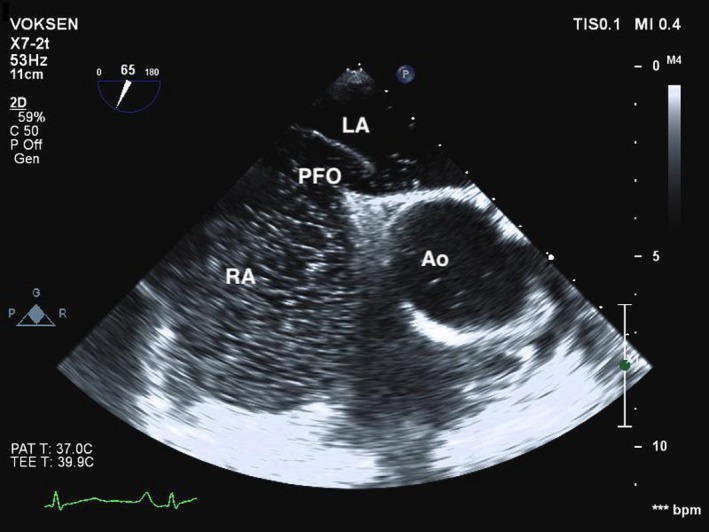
Transesophageal echocardiography verified a large PFO. Ao, aorta; LA, left atrium; PFO, patent foramen ovale; RA, right atrium
